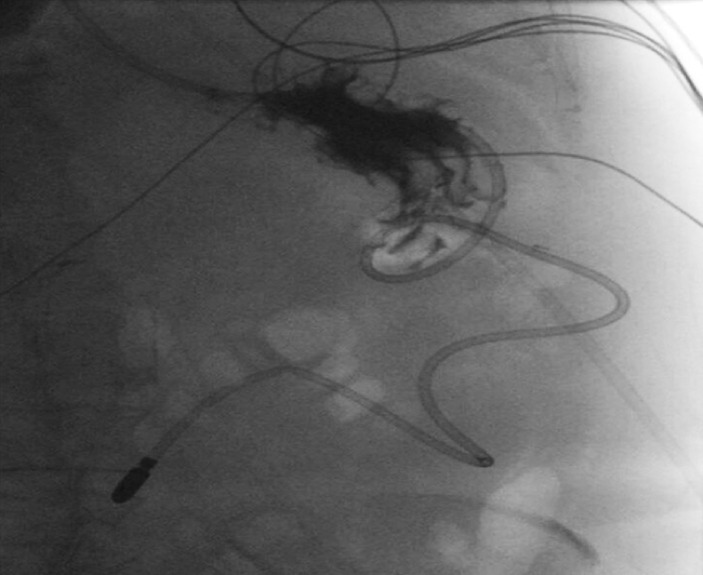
Radiographic image from an upper gastrointestinal series showing no evidence of contrast extravasation, indicating no leakage, and the nasojejunal tube in the efferent limb
